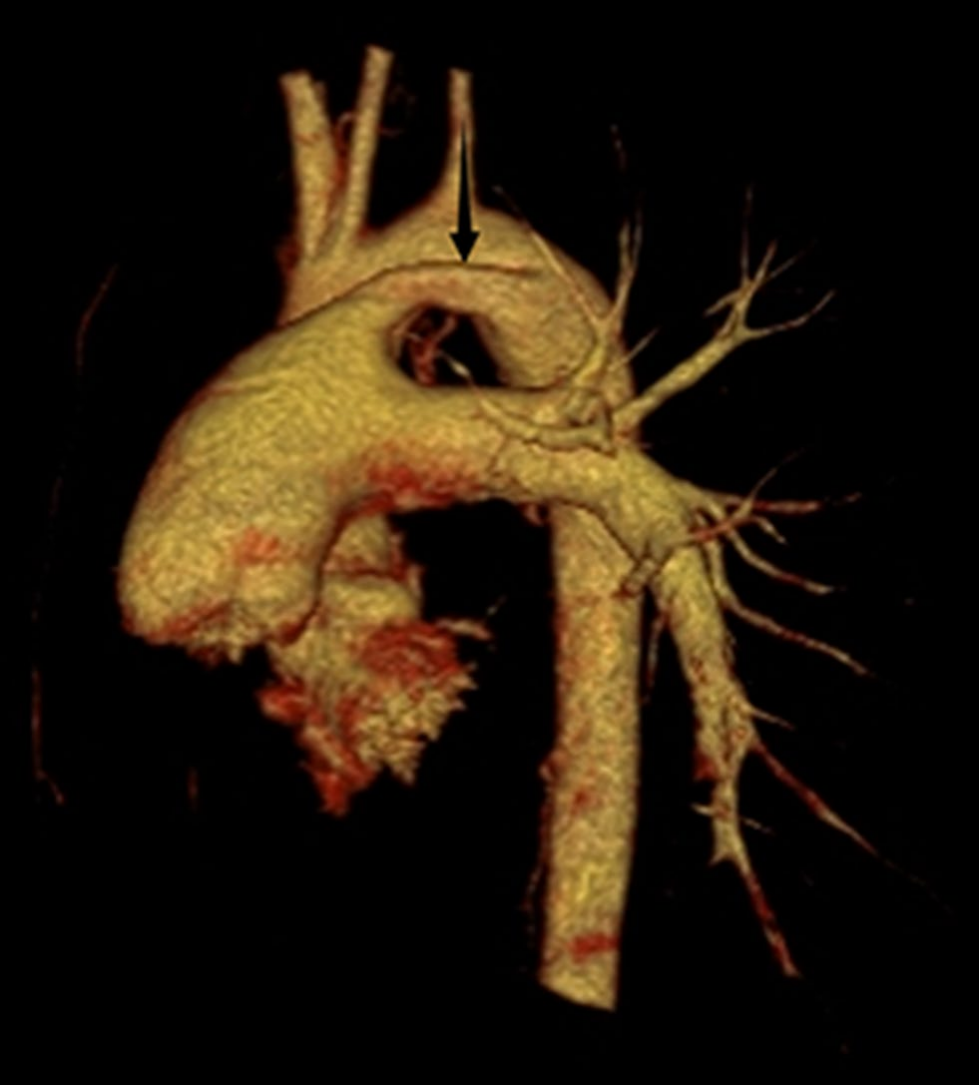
The chest CT scan with three-dimensional reconstruction of a 13-year-old female revealed the absence of the right pulmonary artery (arrow) and patent ductus arteriosus
